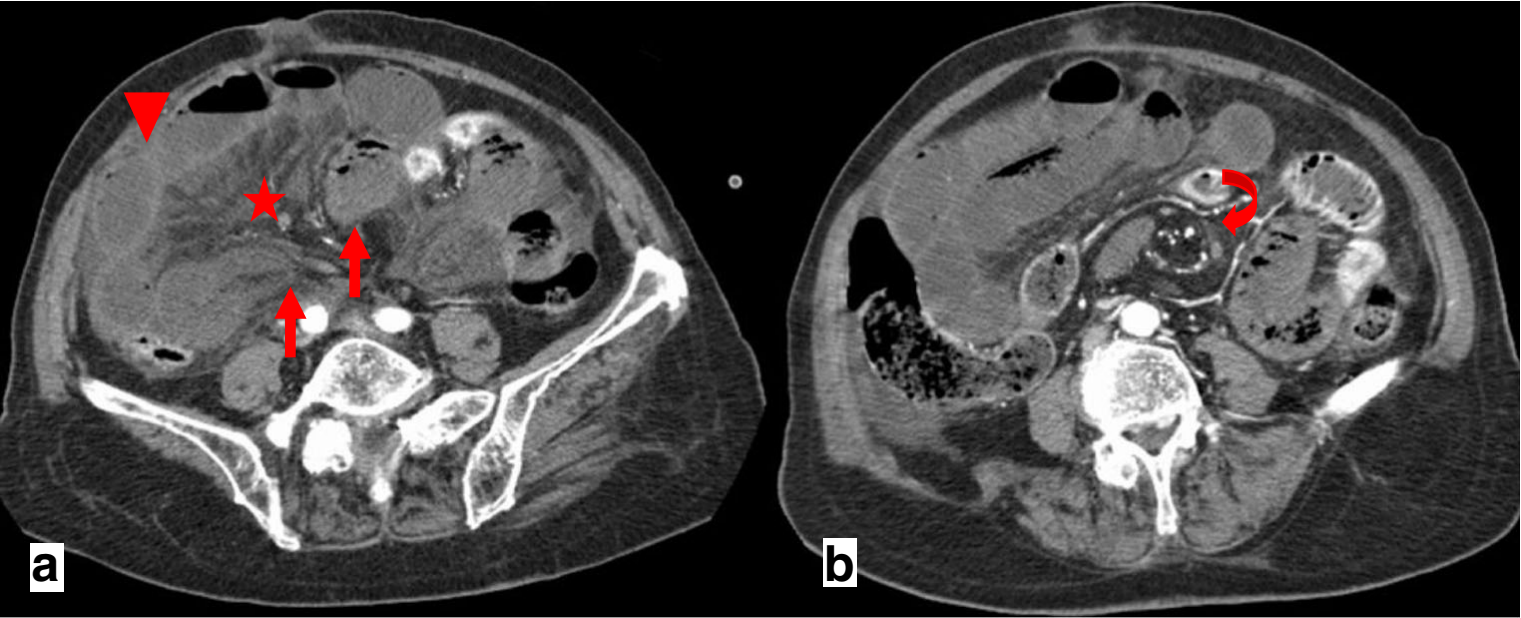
a, b Patient with abdominal pain because of a closed-loop SBO. Both ends of a fluid-filled distended closed loop taper fusiformly, in a way similar to a beak—the beak sign (*arrows in a*). Mesenteric edema (*star in a*), peritoneal fluid and reduced enhancement of the bowel wall comparatively to other small-bowel loops (*arrowhead in a*) are also evident, findings that suggest strangulation. A slightly cranial image shows the twisting of the mesenteric vessels at the mesenteric root—whirl sign (*curved arrow in b*)The whirl sign, which corresponds to the whirled appearance of the mesenteric vessels in the middle of the obstruction point (Fig. 2). The tightness of the whirl pattern reflects the degree to which the mesentery and vessels are rotated. Although hampered by low sensitivity (60 %), a recent study has shown that if this sign is present on a CT scan, a patient is 25 times as likely as a patient without it to undergo surgery [13, 14].A radial distribution, which refers to stretched mesenteric vessels converging toward the central point of obstruction (Fig. 3).
